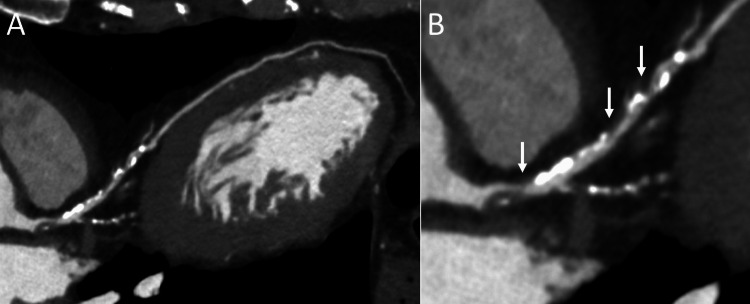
Coronary computed tomography angiography Computed tomography imaging reveals severe coronary stenosis in the left anterior descending artery (A) and an enlarged image of the same artery (B, arrows).
